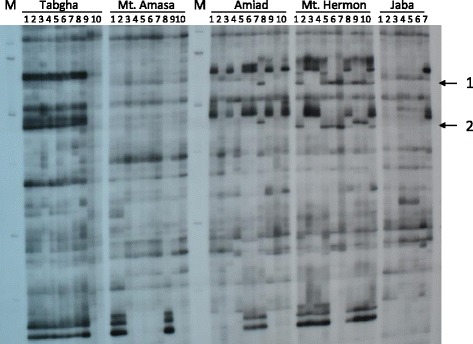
TD gel visualizing insertional patterns of *Aison* (MITE) in 7–10 accessions of 5 wild emmer wheat populations using the *Aison*-specific primer and M-CTA (see Additional file 1: Table S2). Each lane represents one accession and each band represents a single insertion site. M denotes the size marker (100 bp). Examples of population-specific/unique bands are indicated by arrows and numbers: 1 – population-specific insertion (exists in Amiad and Mt. Hermon populations but not in others), 2 – population-unique insertion (exists only in Mt. Hermon)
